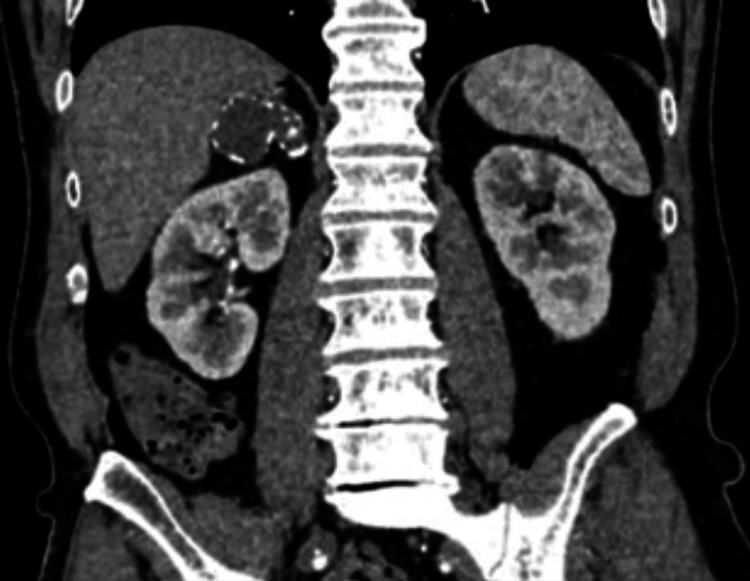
Coronal contrast-enhanced abdominal CT demonstrating a 51×40 mm adrenal lesion with attenuation of 45 HU. The attenuation and enhancement pattern supported a benign etiology and informed preoperative planning. Representative single-patient image; values shown are direct measurements from the depicted case (no statistical comparison performed). CT, computed tomography
